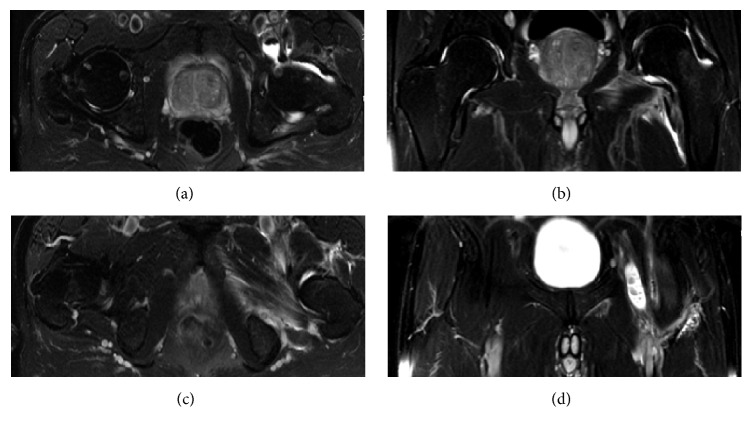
T2 weighted MRI confirms effusion (a, b) with inflammation of the capsule and adjacent muscles and excludes relevant extra-articular abscess formations. Notice the affection of the iliopectineal bursa communicating with the hip joint (a, d).
